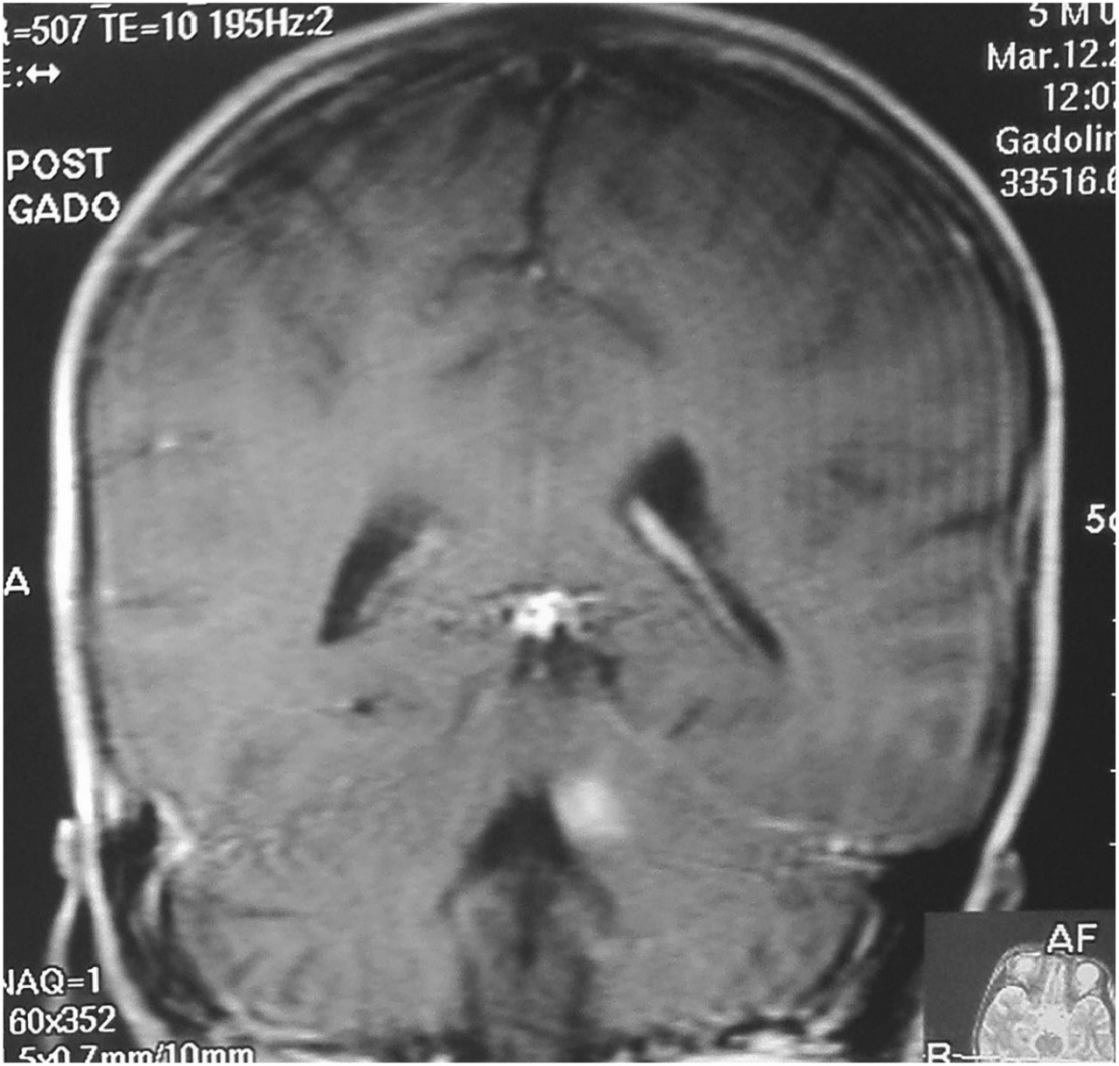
Post contrast MRI of the brain, T1 sequence coronal slice. The scan re‐demonstrates the same lesion seen on the initial CT.
